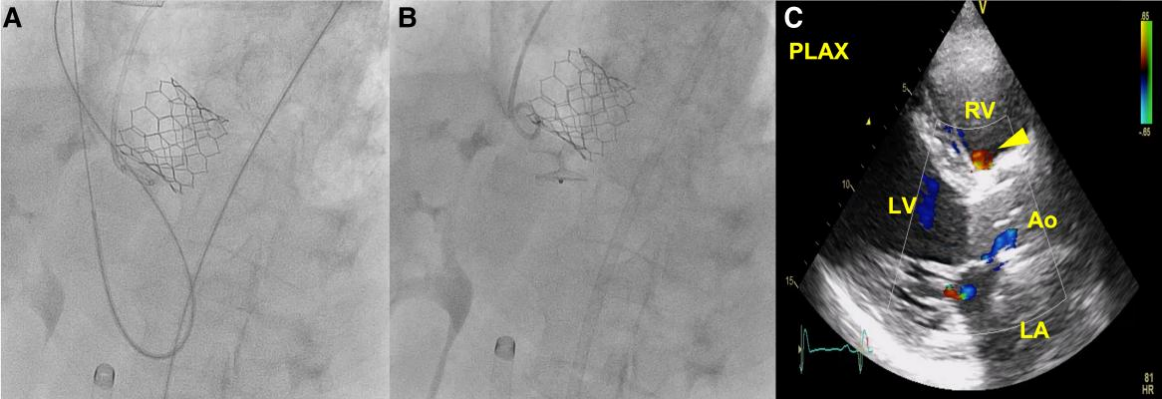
Percutaneous closure of the ARV fistula. (*A* and *B*) Deployment of an 18 mm Amplatzer atrial septal occluder into ARV fistula. (*C*) TTE showing the reduction of the shunt from the aorta root to the right ventricle after Amplatzer deployment (arrowhead). Ao, aortic root; ARV, aorta to right ventricle; LA, left atrium; LV, left ventricle; RV, right ventricle; TTE, transthoracic echocardiogram.
